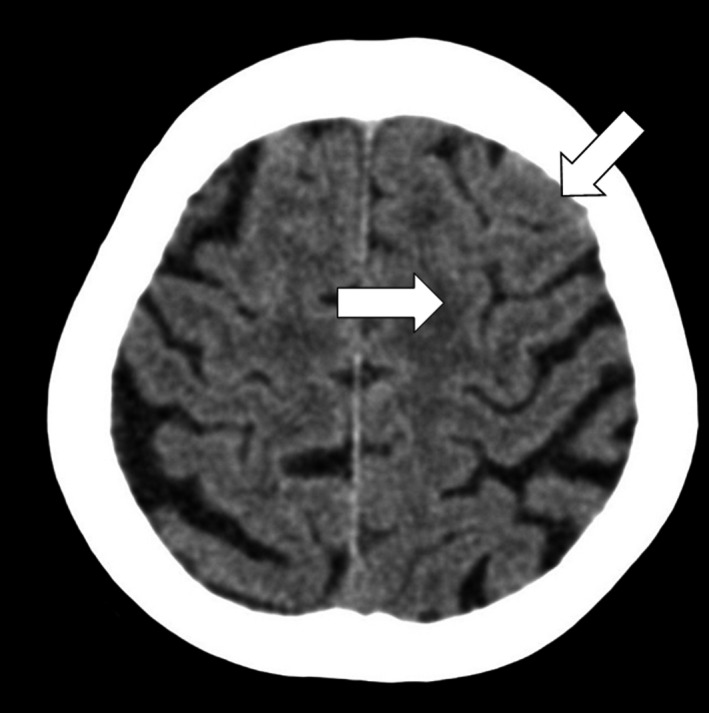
Head CT showed no high densities of left cortical frontal sulci or new lesions (arrows)
